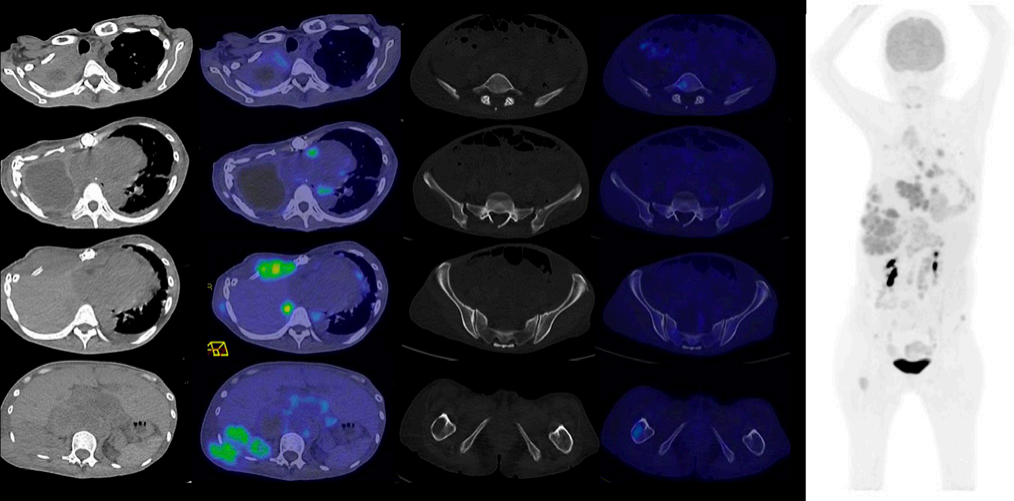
18FDG-PET CT Rainbow axial images (Left, top to bottom) of right apical pleura, mediastinal adenopathy, right anterior chest wall, right posterior chest wall and retroperitoneal adenopathy, (Middle, top to bottom) fifth lumbar vertebra, left ilium, left iliac crest and right hemisacrum, right femur and Maximal Intensity Projection image (Right) after two cycles of PRRT.
